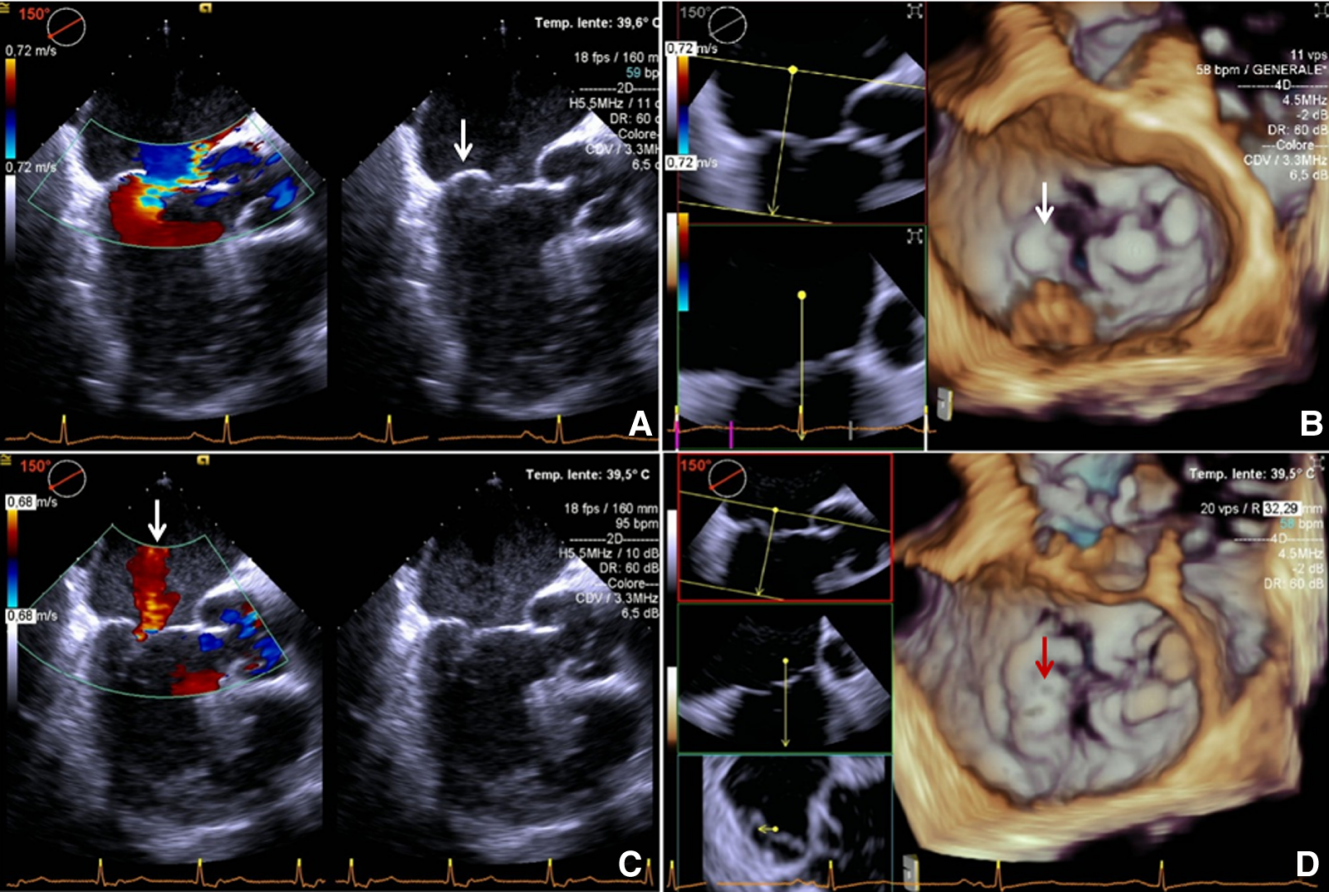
Intraoperative transesophageal echocardiography. (A,B): before retensioning procedure 2D + color Doppler and 3D; eccentric regurgitation jet directed to AML and reprolapsing PML (arrow). (C,D): after retensioning procedure 2D + color Doppler and 3D: (C) central postprocedural mild MR (arrow) is showed. (D) Mild residual PML prolapse is highlighted (arrow) and a good coaptation between AML and PML is reached. AML, anterior mitral leaflet; MR, mitral regurgitation; PML, posterior mitral leaflet.
